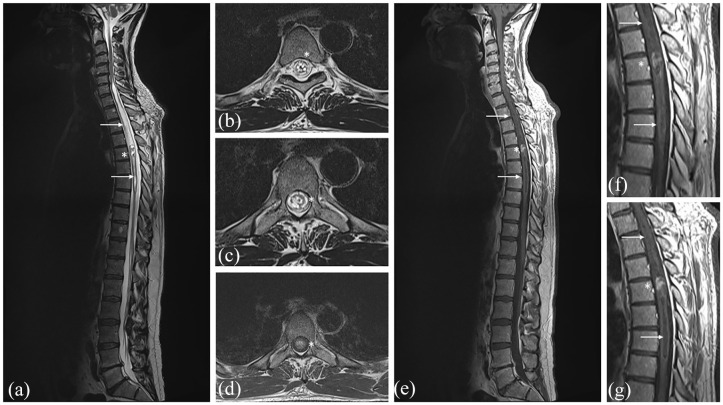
Preoperative T2-weighted MRI showing the intramedullary ependymoma (*) at Th 5 with intertumoral hemorrhage and edema of the spinal cord (→) from C7-Th7 (a-c). T1-weighted MRI with contrast showing the contrast enhancement of the ependymoma (d–g).
